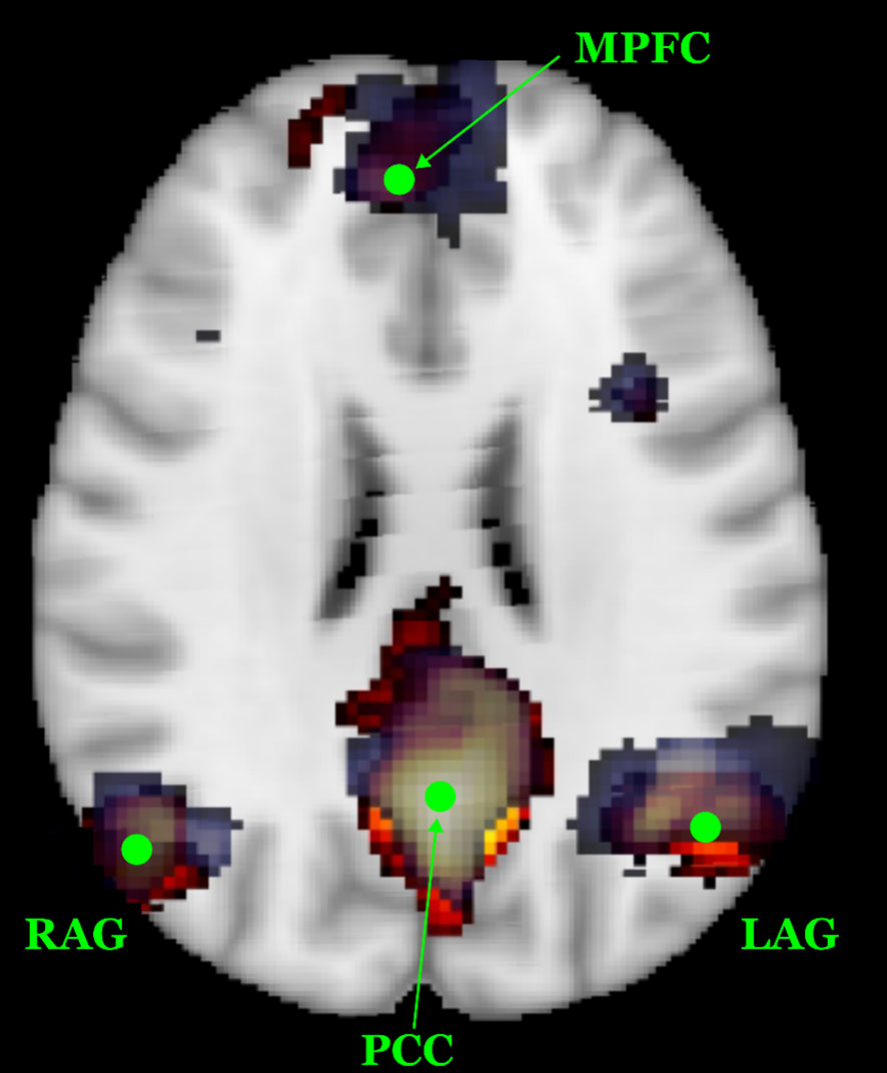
Spatial reproducibility of the DMN topography in the travelling subject across the two MRI sites. The DMN component derived from UO3 is shown on a blue scale, while the component from UO2 is displayed in a red-yellow scale. Green dots indicate the primary DMN regions of interest, i.e. posterior cingulate cortex - PCC, medial prefrontal cortex - MPFC, left and right angular gyri – L/RAG. The substantial spatial overlap highlights good inter-site consistency.
